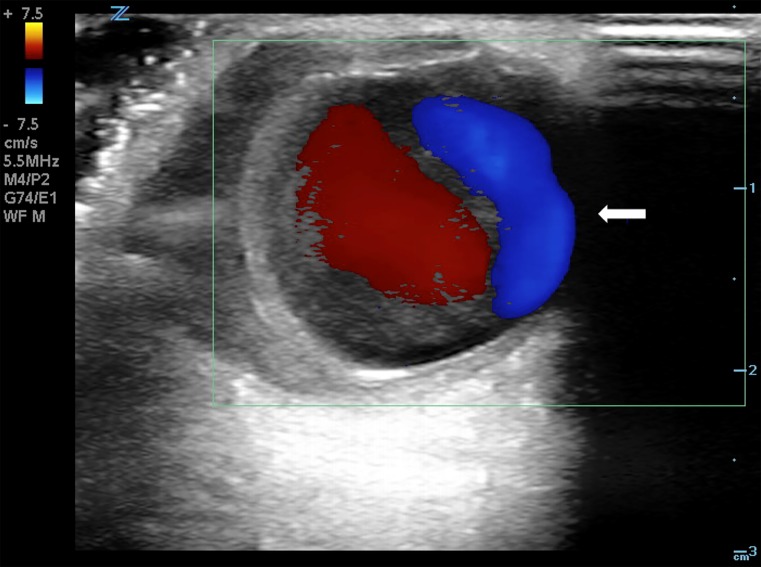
Point-of-care Doppler ultrasound demonstrating the classic “yin-yang” sign (arrow), indicating bidirectional blood flow within the pseudoaneurysm.
